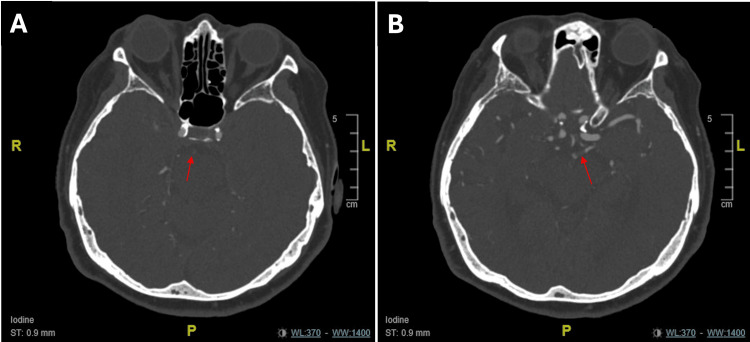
CT angiography of the head (A) Red arrow shows the absence of contrast opacification of the basilar artery, consistent with acute basilar artery occlusion. (B) Red arrow shows the absence of contrast opacification of the P1 segment of the left posterior cerebral artery
